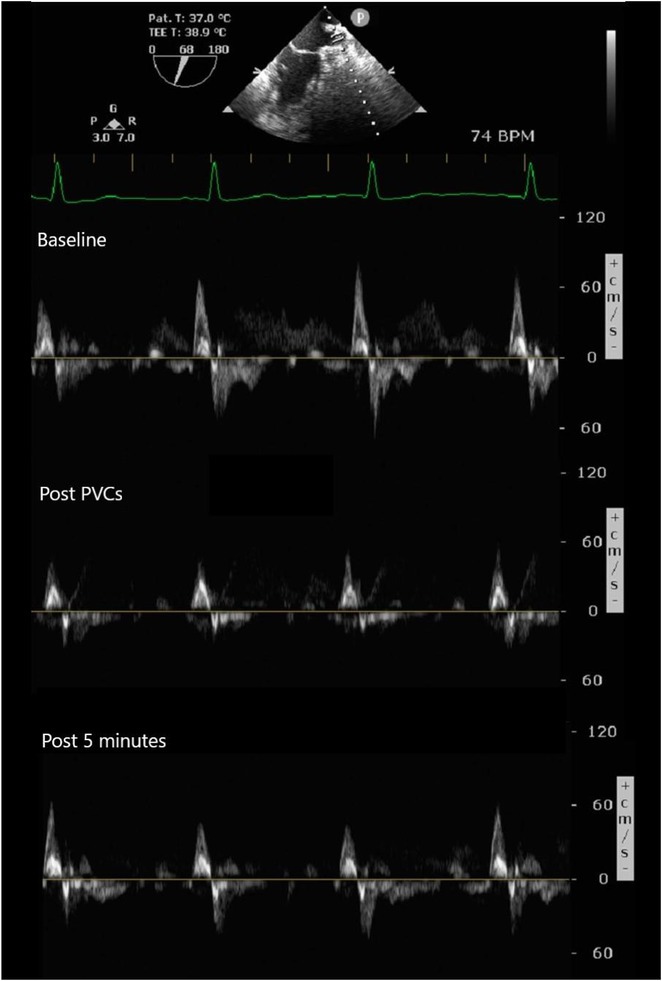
## THE NONLINEAR RELATIONSHIP BETWEEN EPICARDIAL ADIPOSE TISSUE AND ATRIAL FUNCTION IN PAROXYSMAL ATRIAL FIBRILLATION PATIENTS: INSIGHTS FROM CARDIAC MAGNETIC RESONANCE IMAGING
